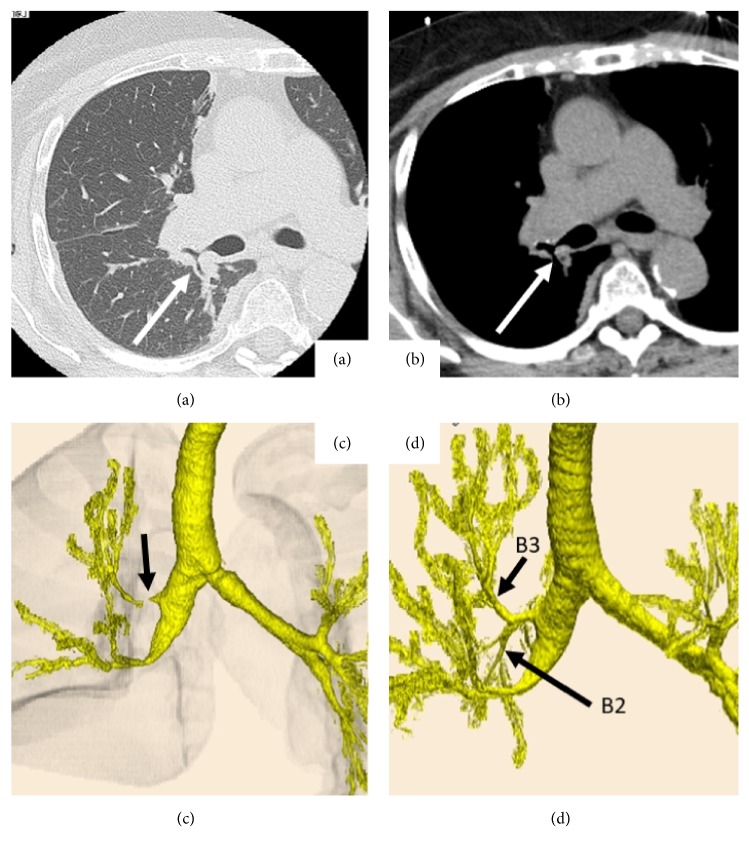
Follow-up chest computed tomography (CT) on postoperative day (POD) 3 with the (a) lung window, (b) mediastinal window, and (c) three-dimensional image revealing improvement of the hilar peribronchovascular soft tissue edema and right upper bronchial stenosis (arrows). (d) 3D-CT on POD 14 showing the counterclockwise rotation of right upper lung lobe and improvement of the right upper bronchial stenosis.
